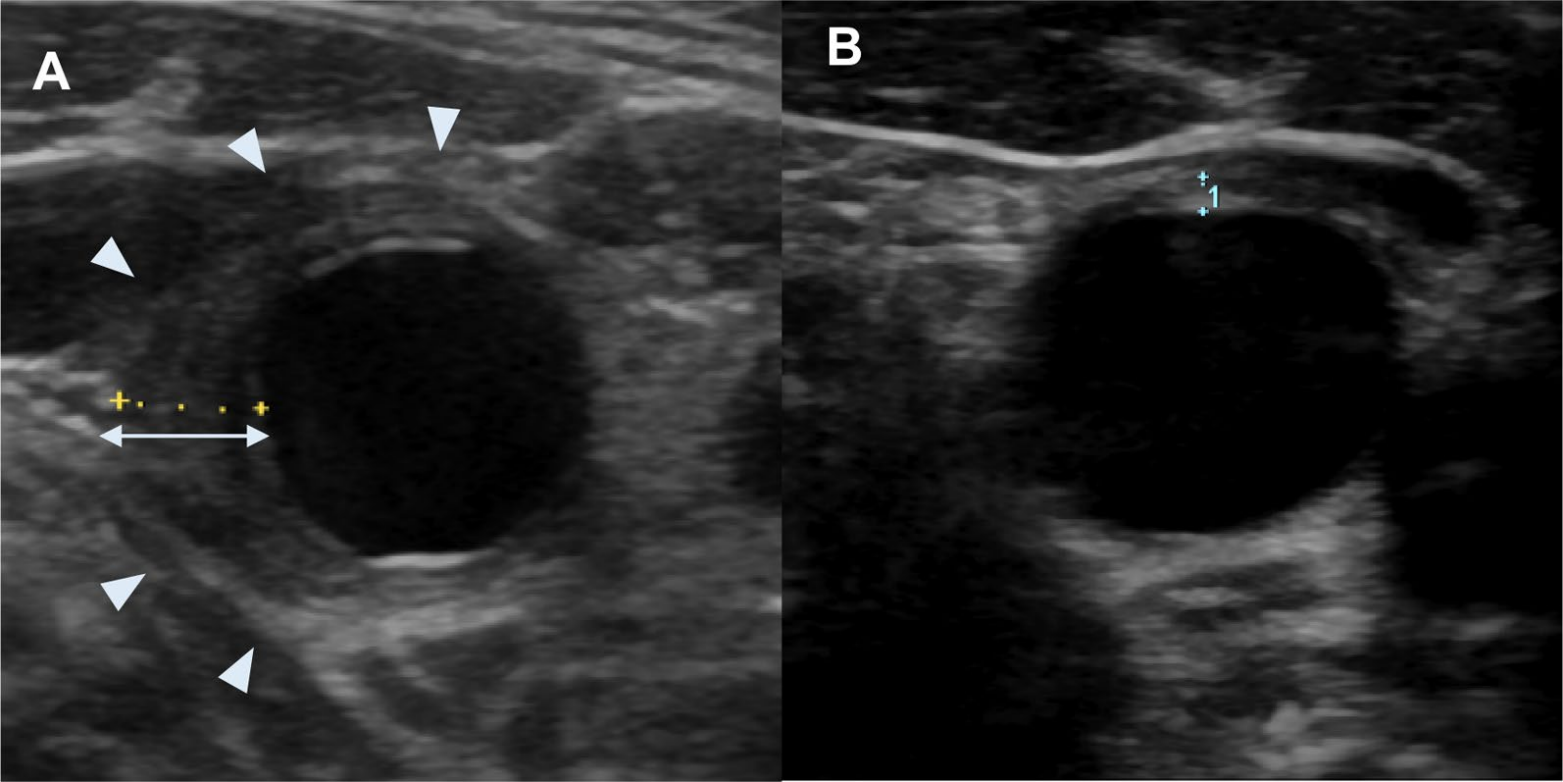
Carotid artery US images before and after treatment. A US image of the right internal carotid artery on day 8 of hospitalization, revealing hypoechoic or isoechoic wall thickening around the vessels from the bilateral common carotid bifurcation to the bilateral internal carotid arteries (white arrowheads). B US image of the right internal carotid artery on day 22 of hospitalization, showing improvement in the vessel wall thickening after treatment. *US* ultrasonography
